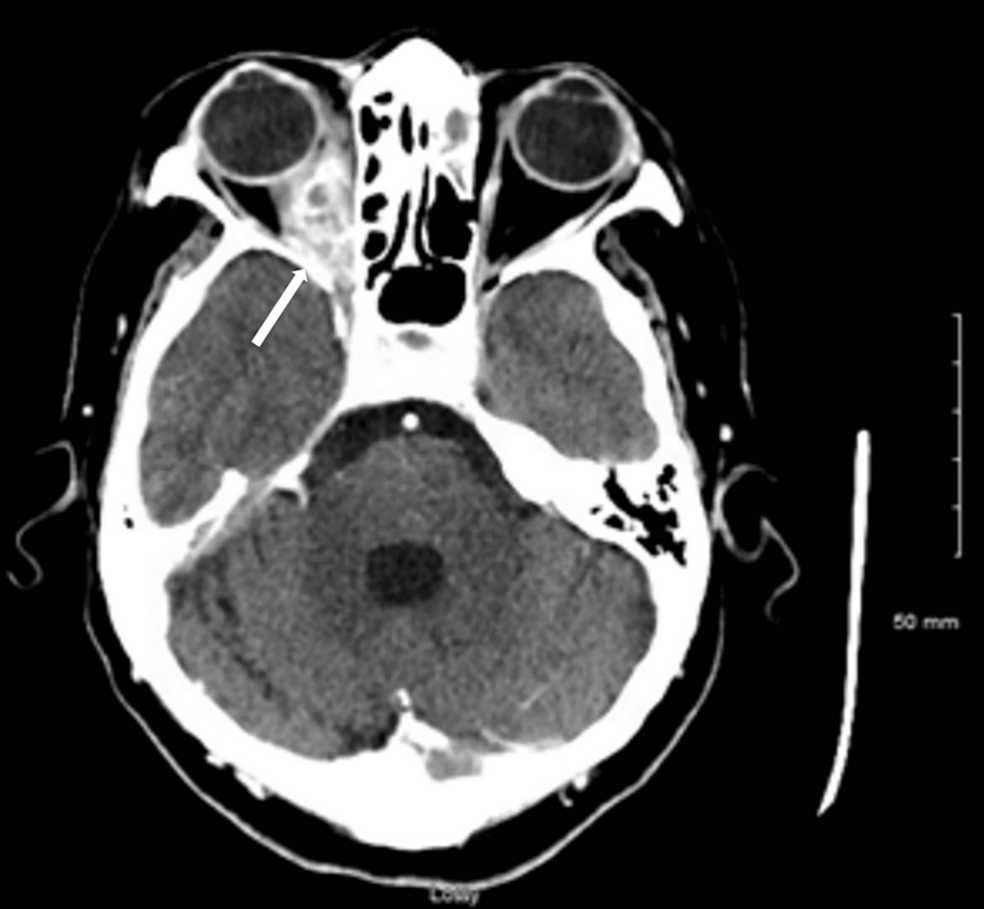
CT of the head demonstrating a 3.5 cm, right orbital, medial wall abscess extending into the orbital apex
